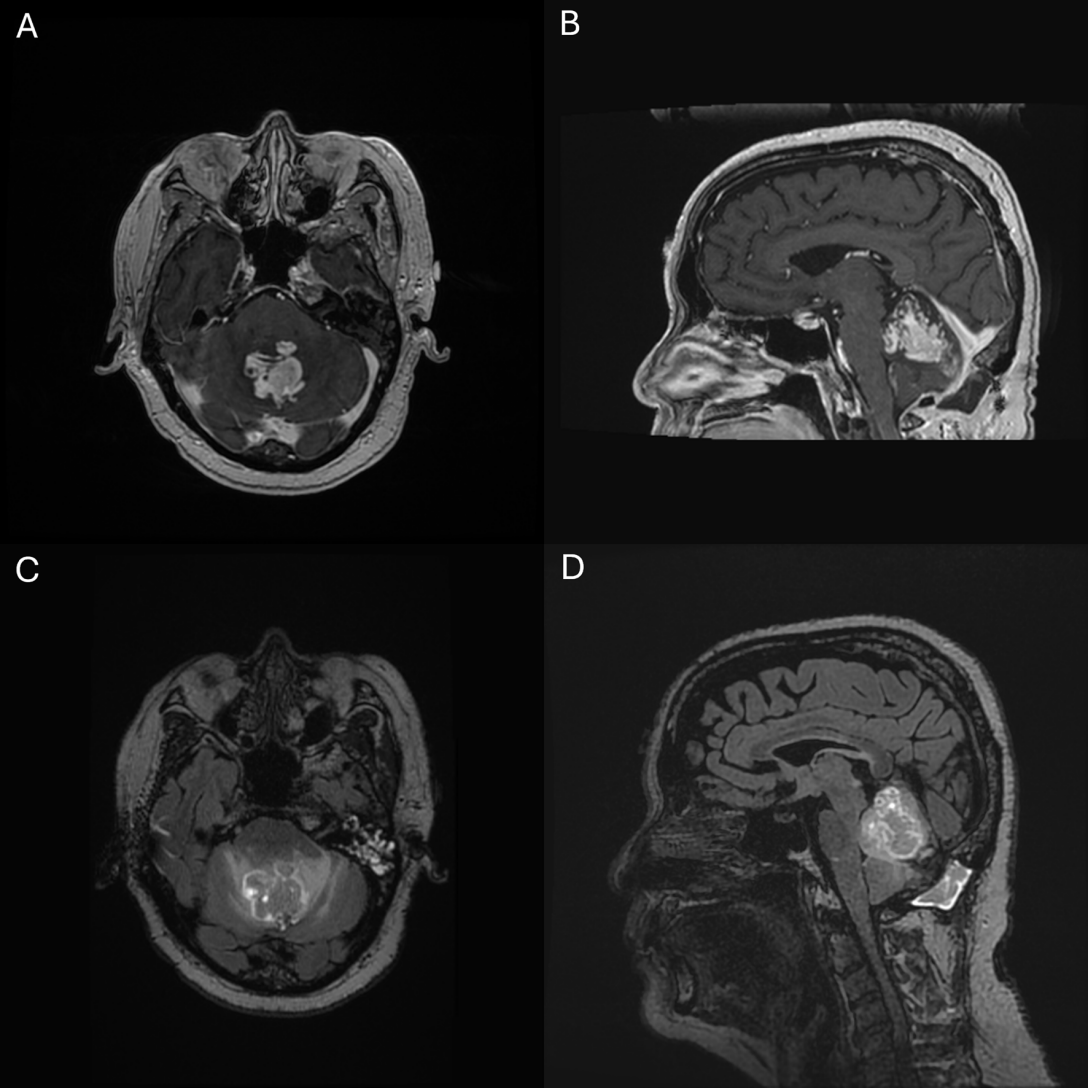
T1 post-contrast (A) axial and (B) sagittal and T2 FLAIR (C) axial and (D) sagittal magnetic resonance images of vermian HGAP in a 68-year-old man. The mass and surrounding cerebellar folia display marked enhancement, suggesting leptomeningeal spread
